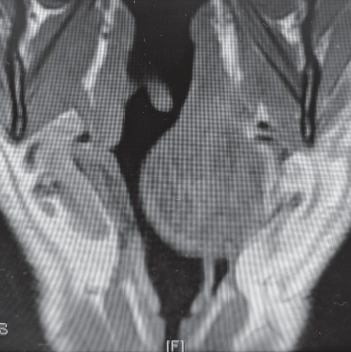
View of mass on axial CT.
